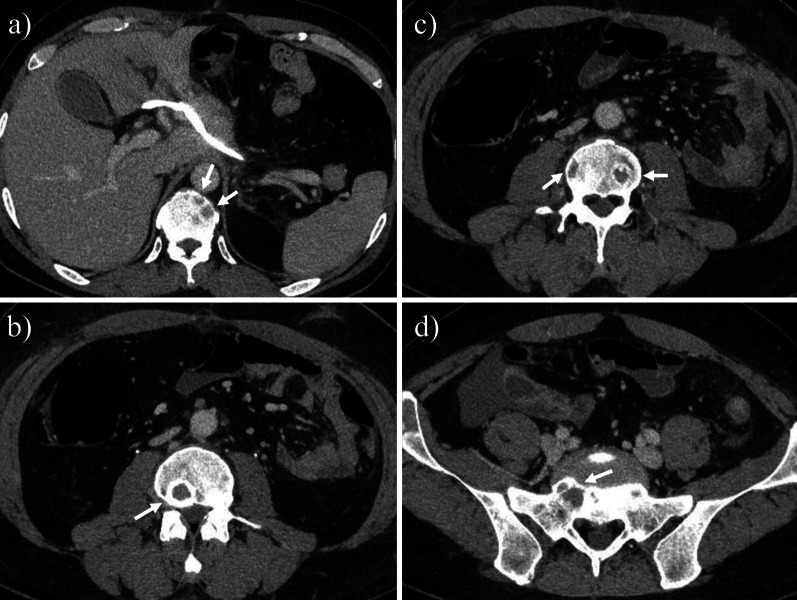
Postoperative CT. Multiple, widespread, low-density masses were seen in the vertebrae (arrow)
